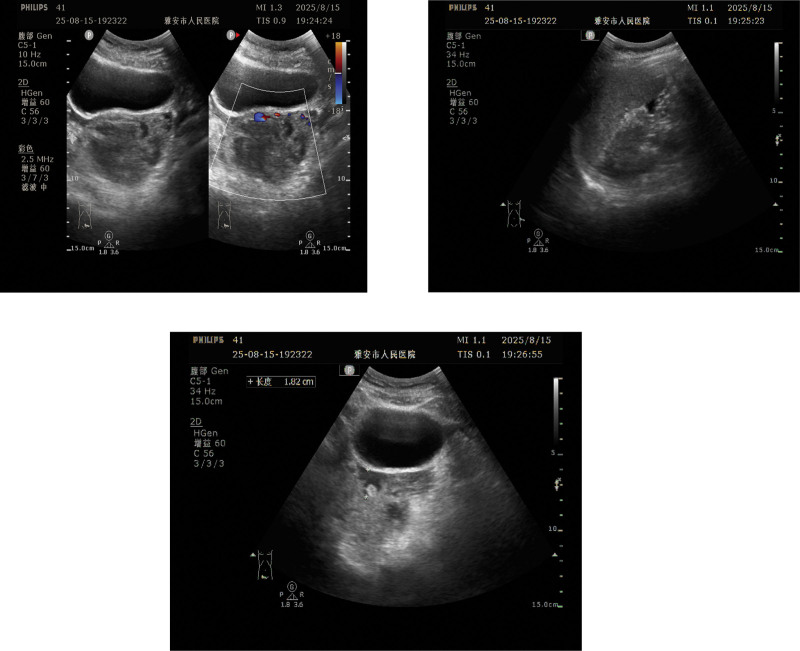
Emergency bedside ultrasound in our hospital showed a normal-sized uterus without a gestational sac in the uterine cavity. A 6.6 cm × 6.3 cm × 5.5 cm mixed-echo mass was detected in the left adnexa, with ill-defined boundaries and irregular shape. CDFI showed abundant blood flow signals around the mass. A large area of hypoechoic region (about 12 cm × 8 cm) was seen in the abdominal cavity, suggesting acute intra-abdominal hemorrhage. CDFI = color Doppler flow imaging.
